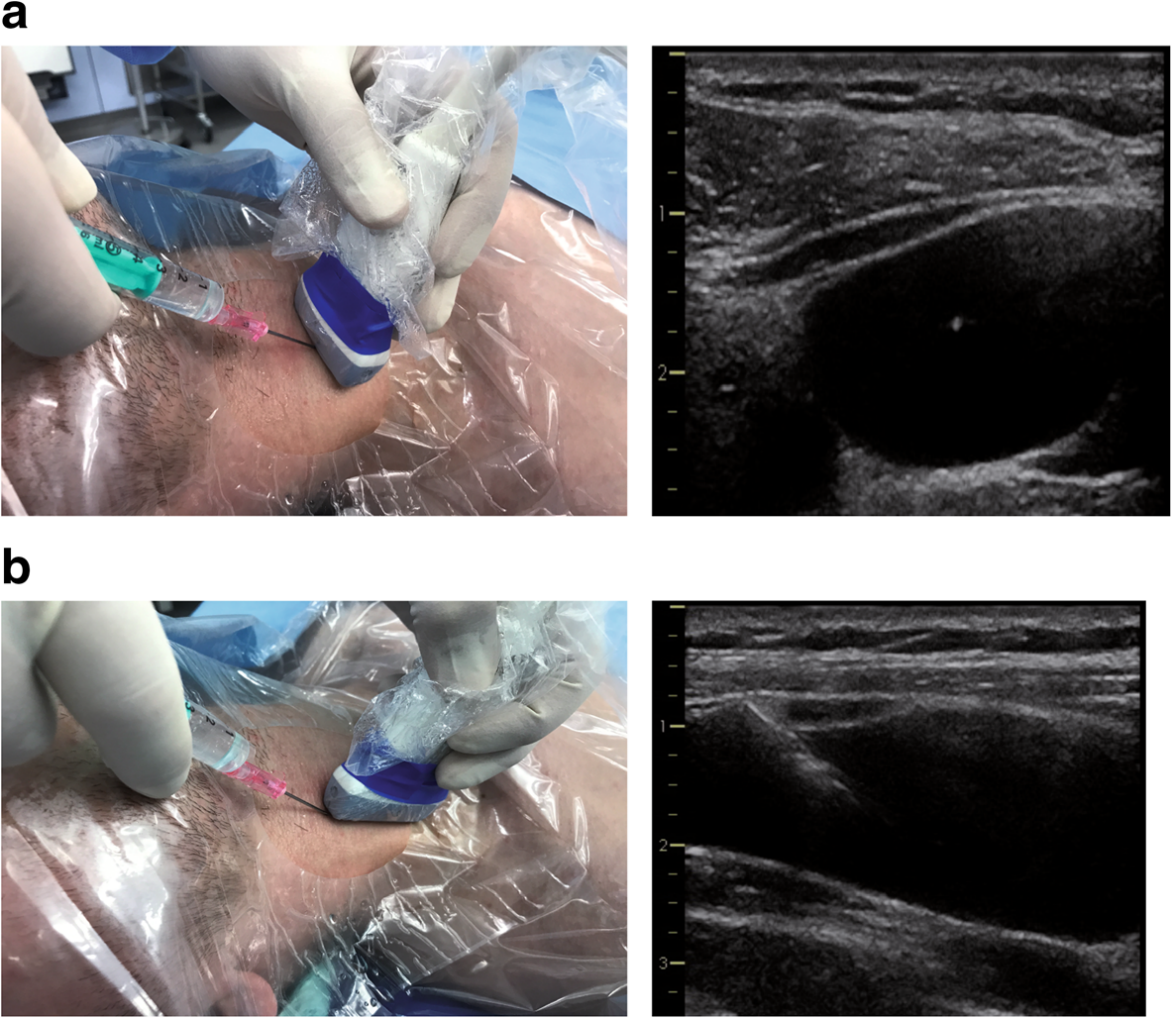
Ultrasound probe orientation and view of the needle. Ultrasound guidance during needle advancement can be performed using a short-axis probe orientation and an out-of-plane view of the needle (a) or a long-axis probe orientation and an in-plane view of the needle (b)
